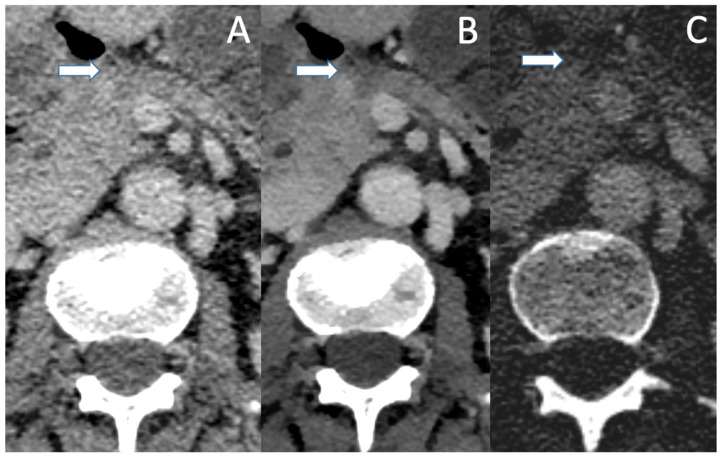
A patient with PDAC after neoadjuvant chemotherapy in conventional CT (A), VMI (B) and ID (C) in the portal venous phase. The small residual tumor (arrow) is barely visible in the conventional image and no statement about vascular association can be made. VMI and ID show a well-demarcated tumor which shows contact with the VMS, with no clear evidence of vascular infiltration.
